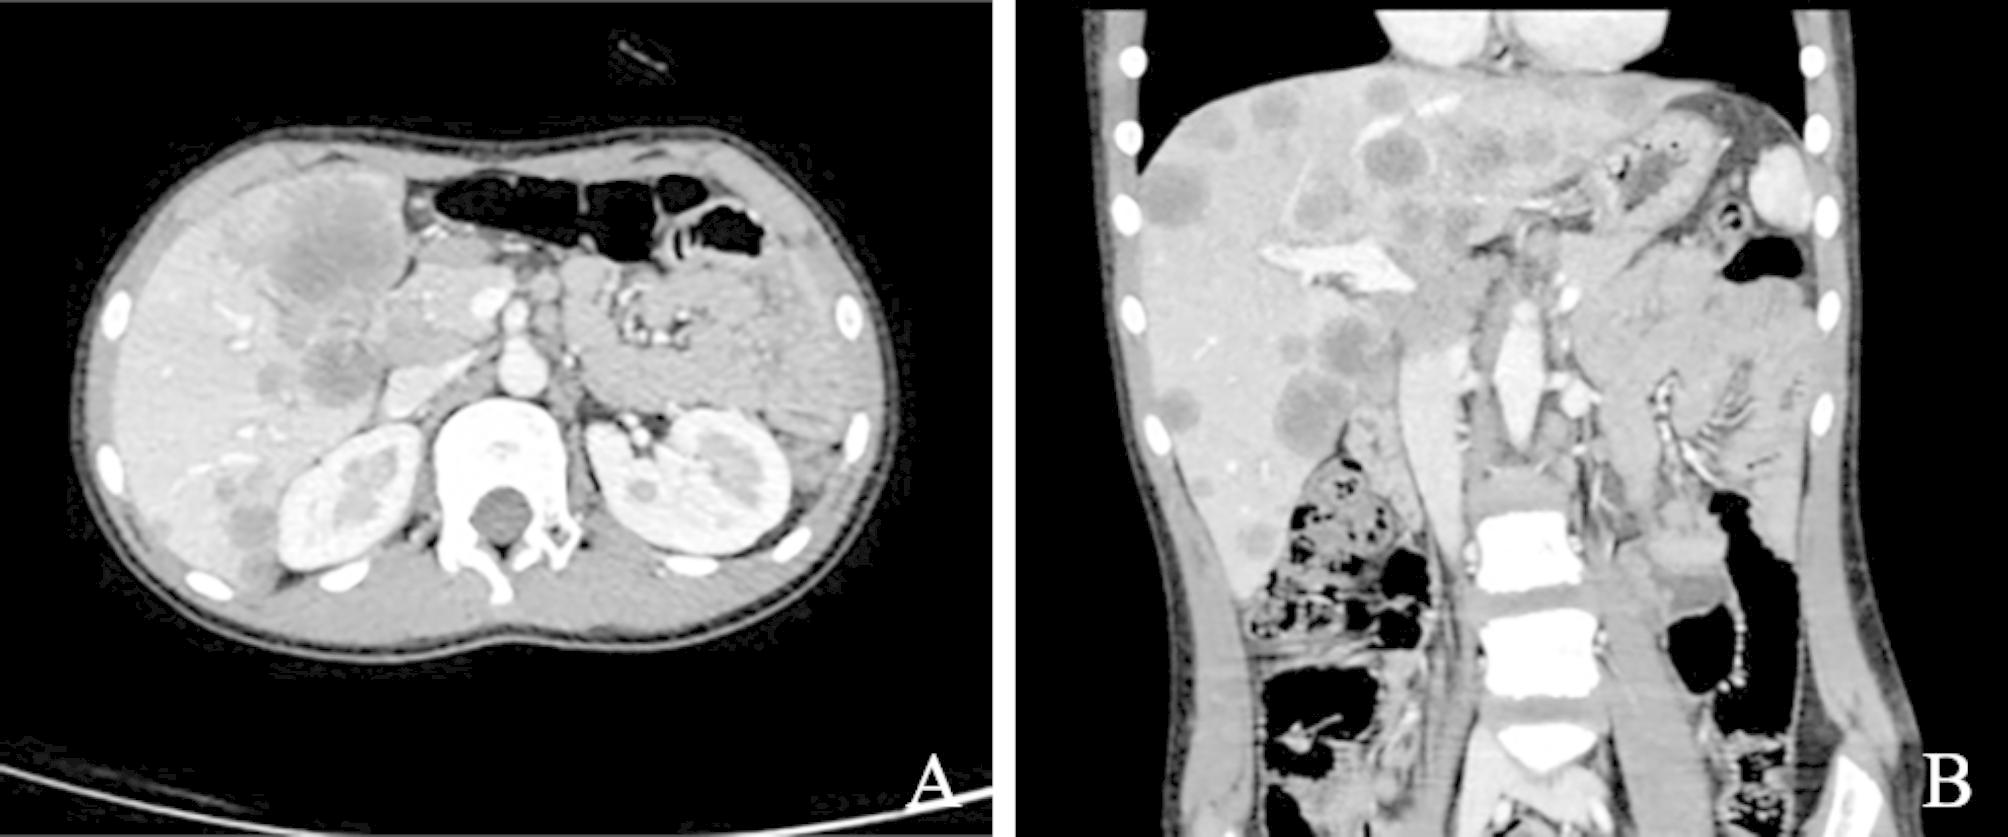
A and B, CT scan showed multiple low-density masses in the liver, the largest of which was located in the lower part of the left lobe of the liver (indicated by the white arrow), where A was cross-sectional and B was sagittal
